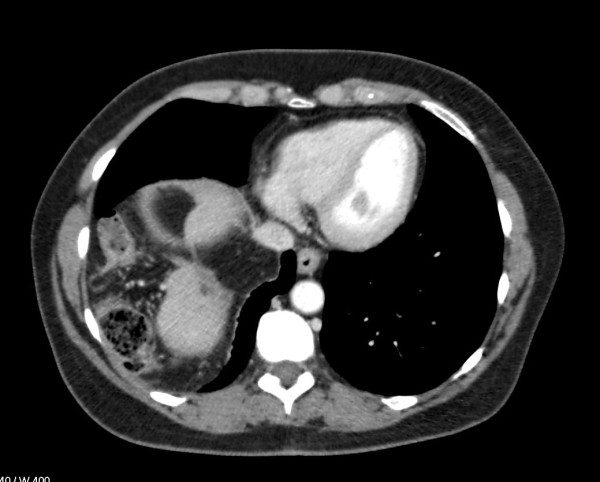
CT scan Transversal computed tomography (CT) showing the loop of colon in the right-sided diaphragmatic hernia.
